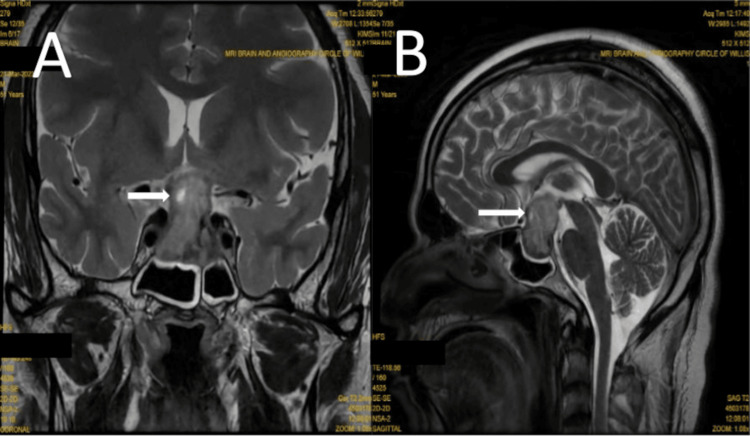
Case 4: T2-weighted MRI image showing pituitary apoplexy (arrow) in a macroadenoma of size 1.9 cm x 3.3 cm x 3.4 cm (anteroposterior x transverse x craniocaudal). (A) coronal section and (B) sagittal section.
